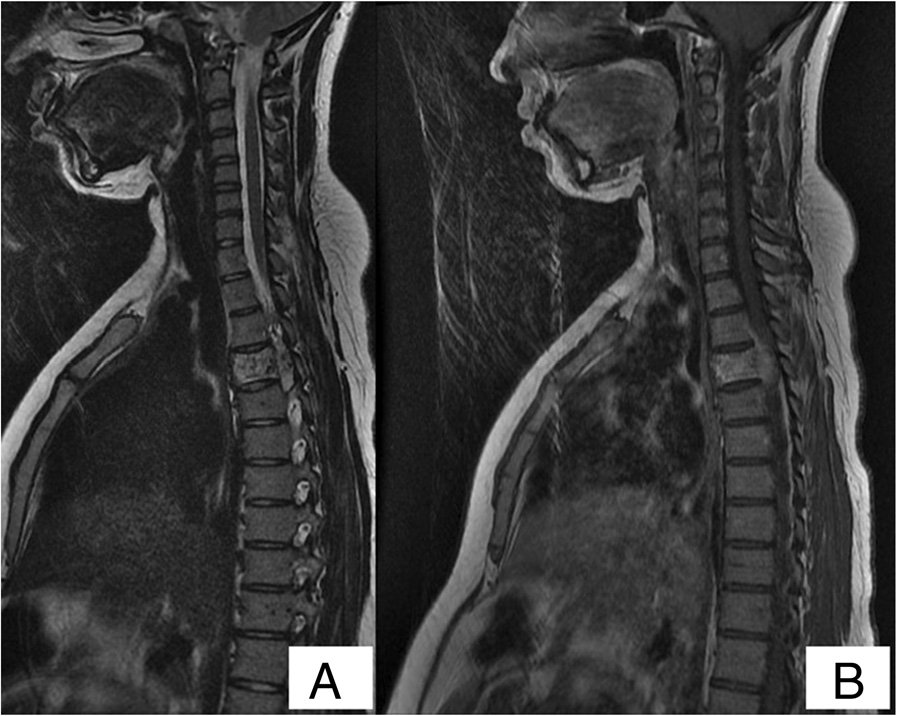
Lateral tomography radiographs. (A) T2-weighted magnetic resonance image and. (B) T1-weighted magnetic resonance image of the thoracic spine demonstrating the lesion in the T4 vertebra (fourth thoracic vertebra).
